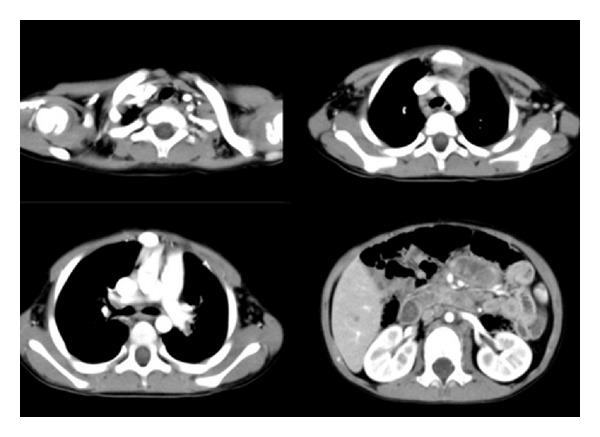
After five weeks of treatment, the thoracic and abdominal CT showed that the swelling of the lymph nodes was markedly diminished.
